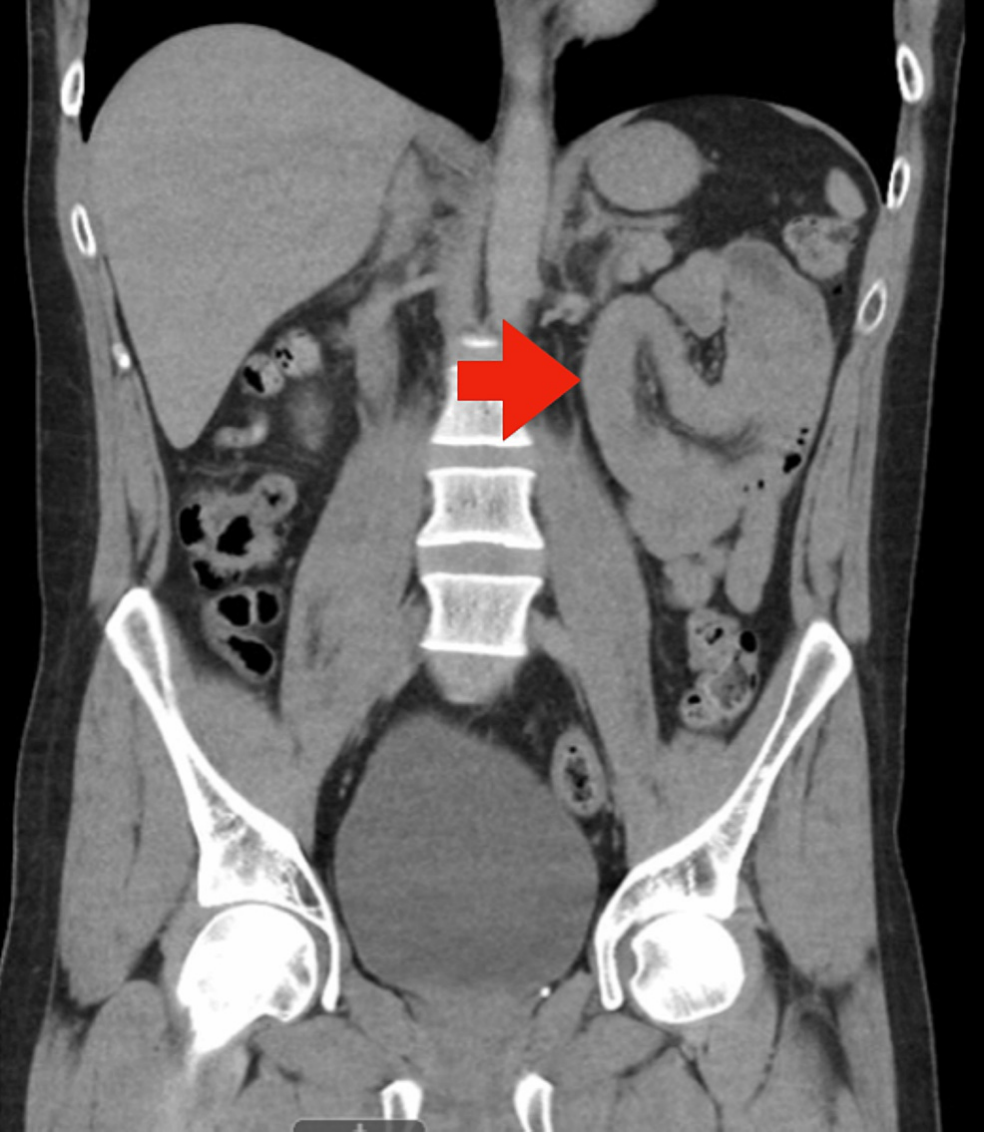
CT abdomen/pelvis without contrast demonstrating intussusception of the proximal jejunum containing a possible round intraluminal mass
